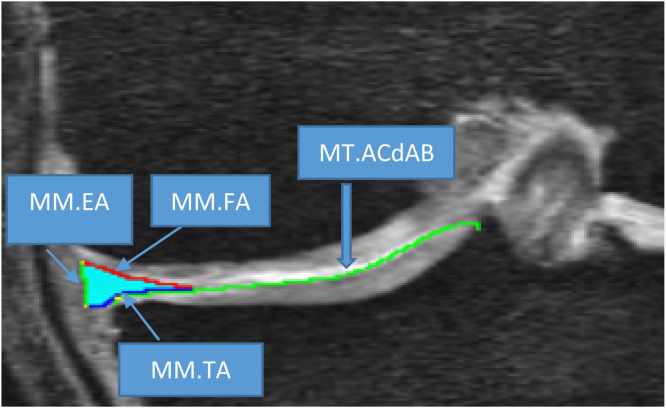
MRI showing manual segmentation of the medial meniscus, highlighting the meniscal areas (TA: tibial area; FA: femoral area; EA: external area) and cartilage surface area (ACdAB) of the MT.
